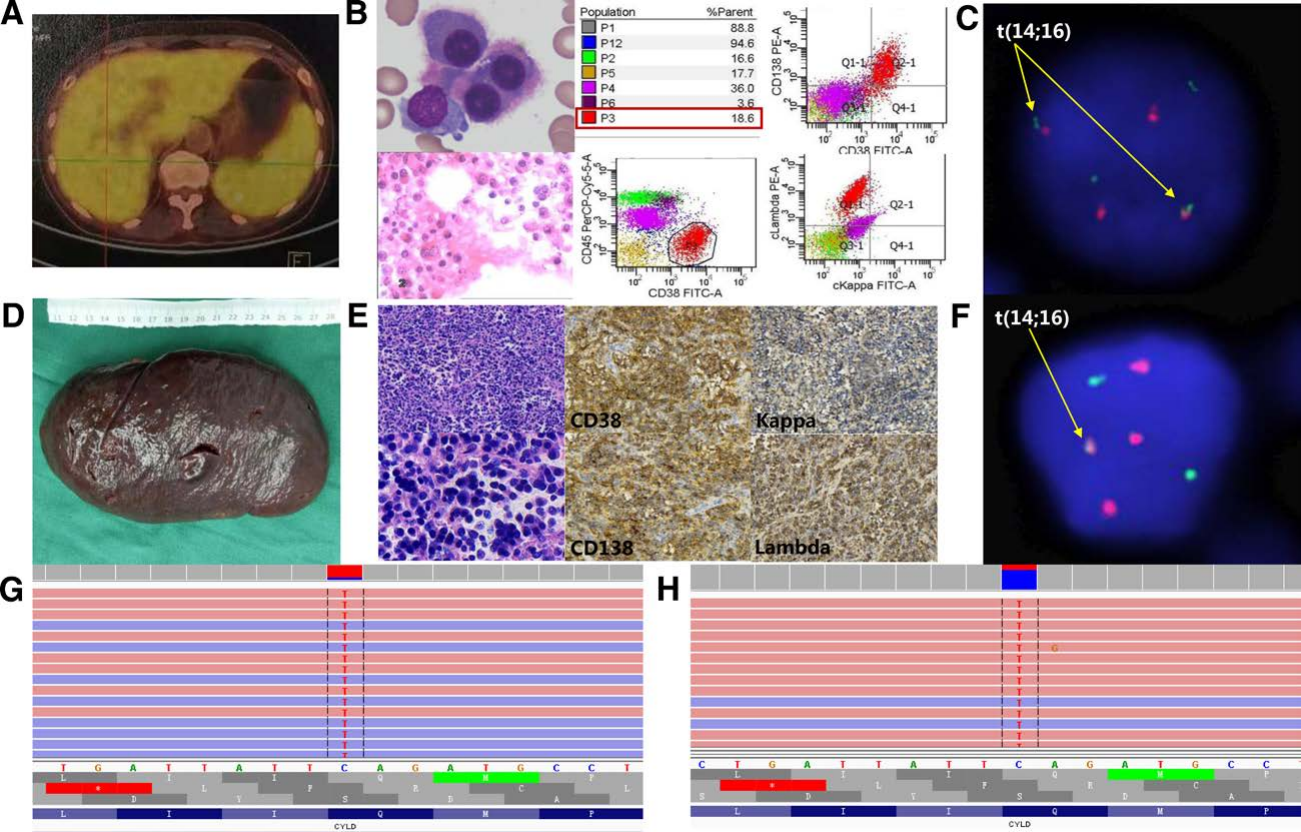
The imaging, bone marrow and spleen examinations of the patient. (A) Enlarged liver and spleen with increased FDG uptake (SUV of 5.3) revealed by PET-CT. (B) Malignant plasma cells in bone marrow detected by morphology, FCI and biopsy. These PCs mainly expressed CD38, CD138, cLambda and were negative for cKappa. (C) Rearrangement and amplification of the MAF gene (IgH/MAF Dual-Color Dual Fusion probe) of BM. (D) The resected spleen was as large as 19 × 10 × 5.5 cm. (E) Diffuse infiltration of malignant plasma cells in the spleen. (F) Rearrangement and amplification of the MAF gene (IgH/MAF Dual-Color Dual Fusion probe) of spleen. (G) and (H) A novel point mutation of CYLD (NM_015247: exon17: c. C226 3T: p.Q755X) of BM (G) and spleen (H) discovered by NGS.
